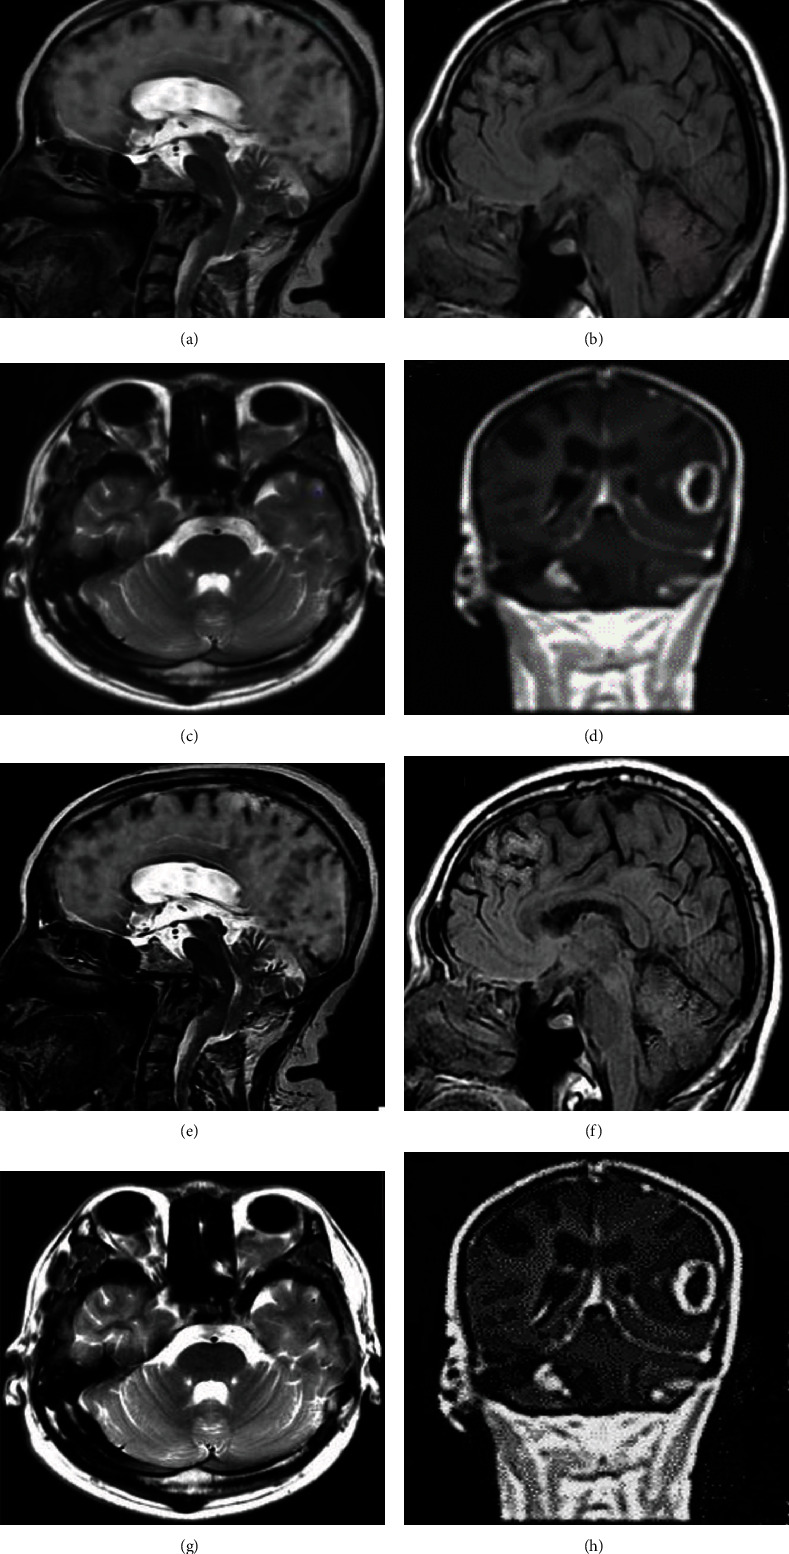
FCM algorithm denoising results. ABCD showed the patient's head MRI image, and EFGH were the images realized by FCM algorithm.
